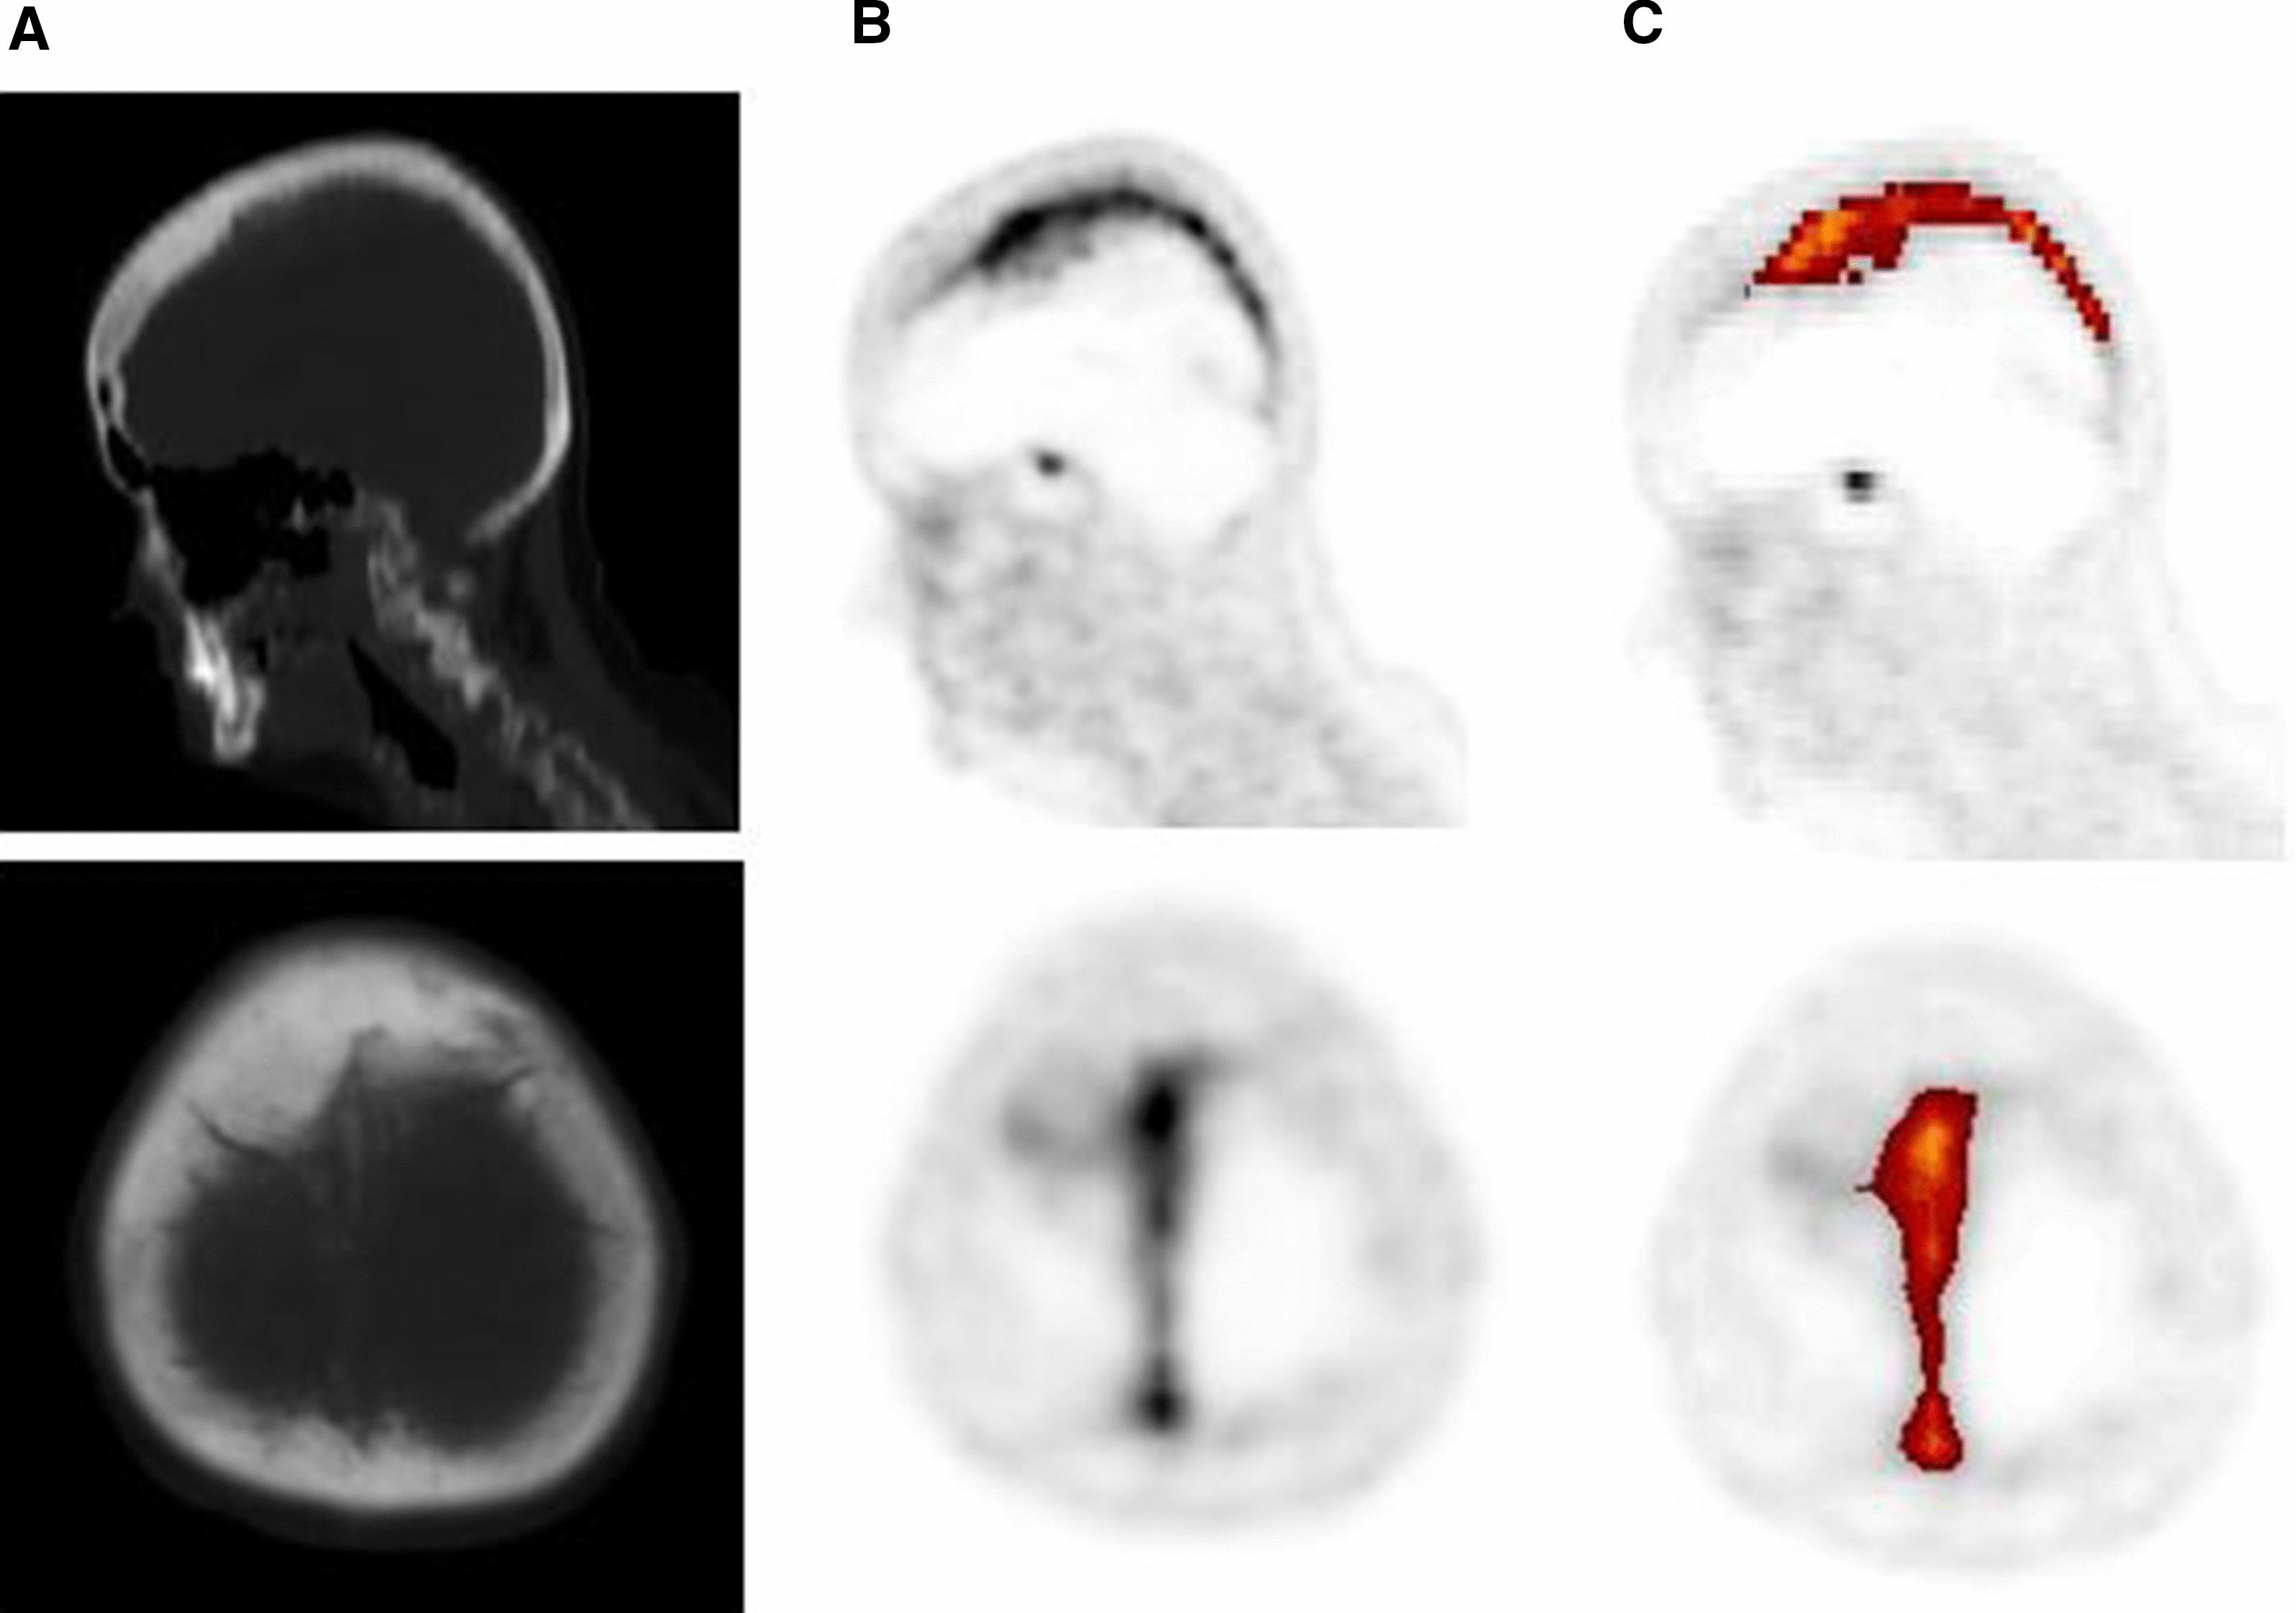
68 Ga-DOTATATE PET highlighting a meningioma with falx involvement. A Sagittal and axial CT-scans, B Sagittal and axial 68 Ga-DOTATATE PET images. C Images from ROVER adaptive thresholding software
